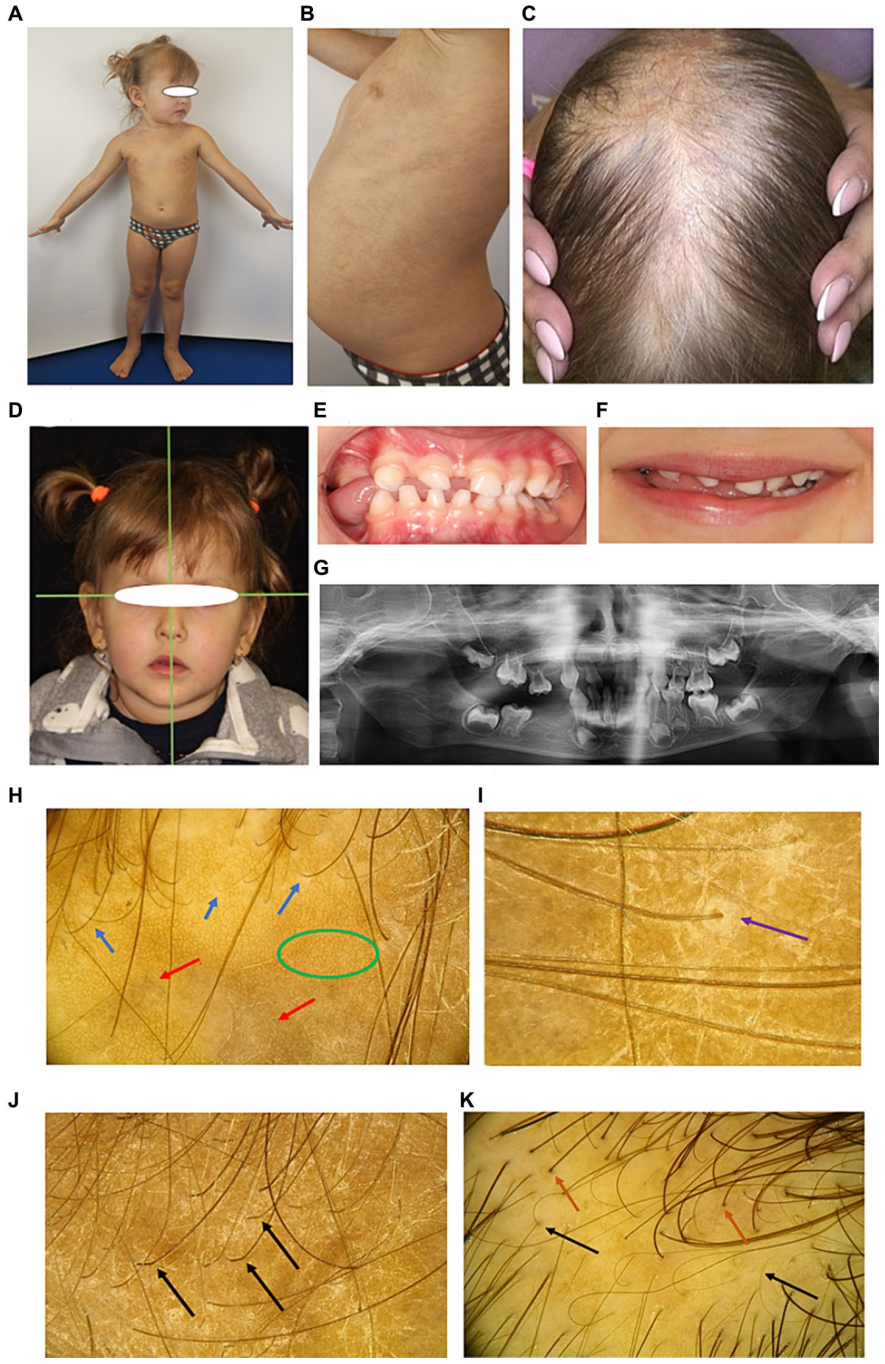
Phenotypic characteristics of proband’s sister S2 (A–G) and trichoscopic hair evaluation of family members (H–K). (A) Overall view. (B) Light brown linear, curved and “star-like” macules are preserved on the trunk. (C) Severe alopecia in parietal zone. (D) Compensatory tilt of the head to the left, corners of the mouth downwards, lips are not closed in the resting position. (E) Laying of the tongue between the teeth on the right side in the area of missing teeth. (F) The smile is asymmetrical, the tongue is inserted between the teeth on the right side. (G) Orthopantomogram reveals multiple tooth adentia. (H) Trichoscopy of the scalp of S1 and S2 demonstrates growth of mostly single hair, white peripilar dots (blue arrows), honeycomb-shaped pigmentation (green oval), and scalp discoloration (red arrows). (I) Keratinous perifollicular desquamation (purple arrow). (J) Boomerang-type hair bending in the proximal zone of growing single hair (shown by arrows). (K) A large number of single growing hair (brown arrows) and the presence of vellus hair (black arrows) on the scalp of M.
